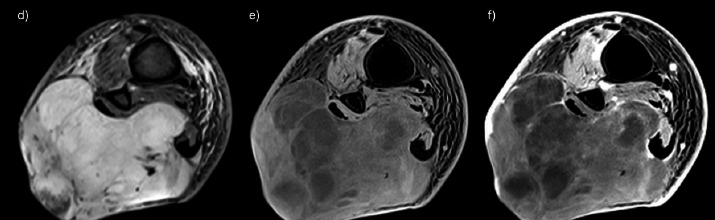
Magnetic resonance imaging (MRI) of the right leg. (a) Axial T2 weighted image. (b) (Coronal Short-Tau-Inversion-Recovery (STIR) image. (c) Sagittal T2 weighted image. (d) Axial Gradient Echo (GRE) image. (e)Axial fat-saturated T1 weighted image. (f) Axial fat-saturated T1 weighted image post-contrast
